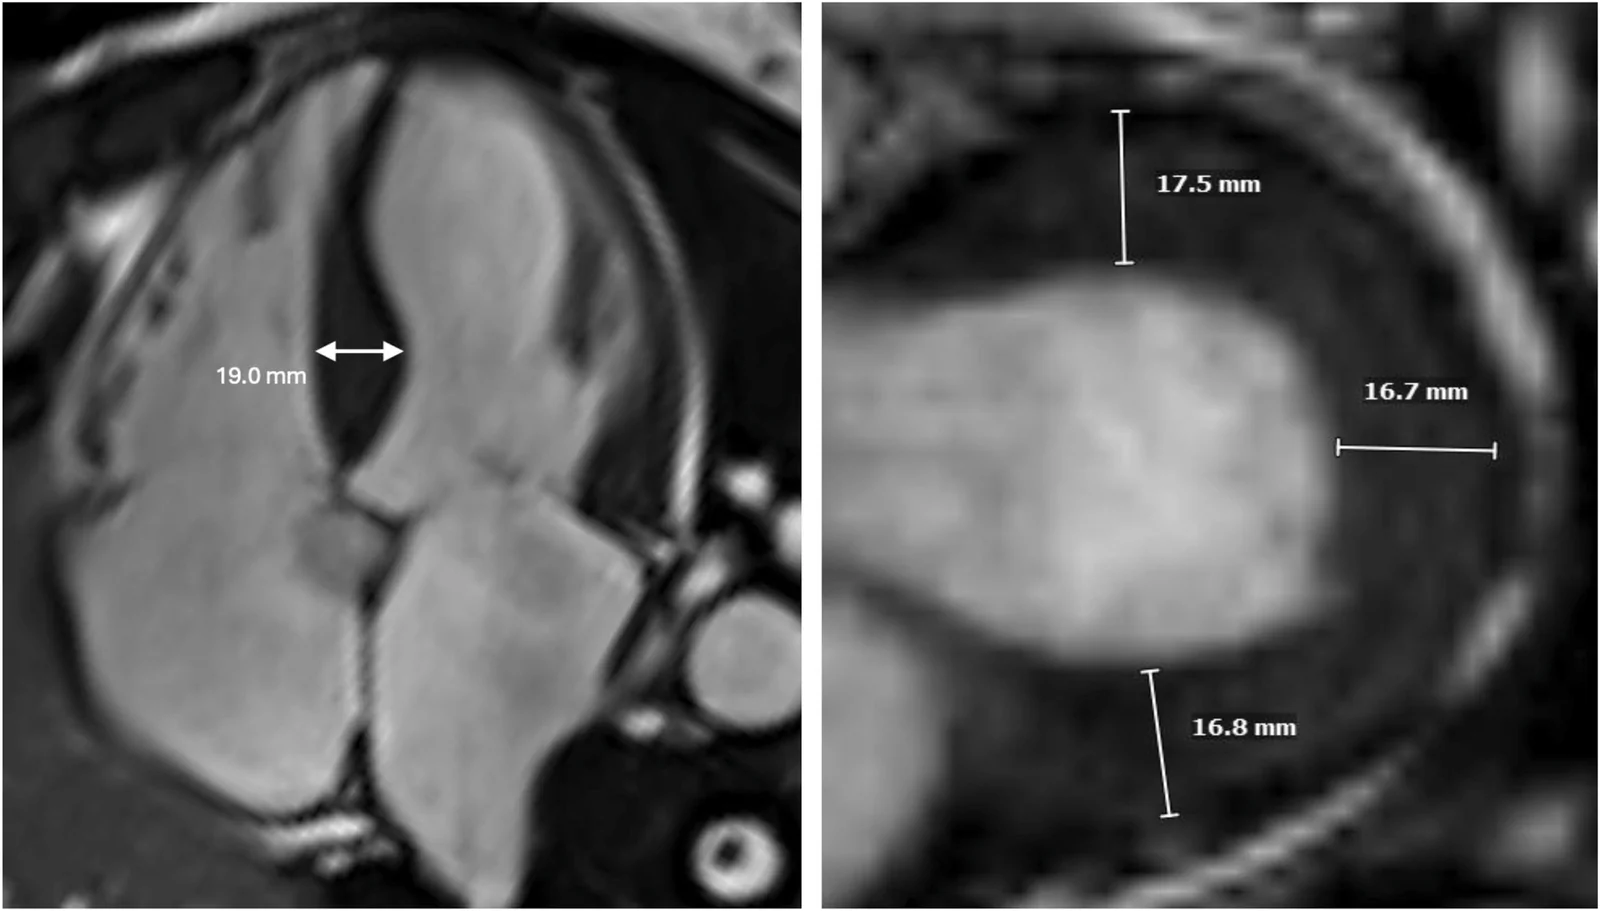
T1-Weighted Cine CMR Images T1-weighted cine (steady-state free precession) images from cardiac magnetic resonance (CMR) demonstrate isolated basal left ventricular wall thickening (19 mm basal septum, 17 mm basal inferolateral wall) with otherwise normal wall thickness in the mid–left ventricular to apical left ventricular walls, right ventricular free walls, and atrial walls.
